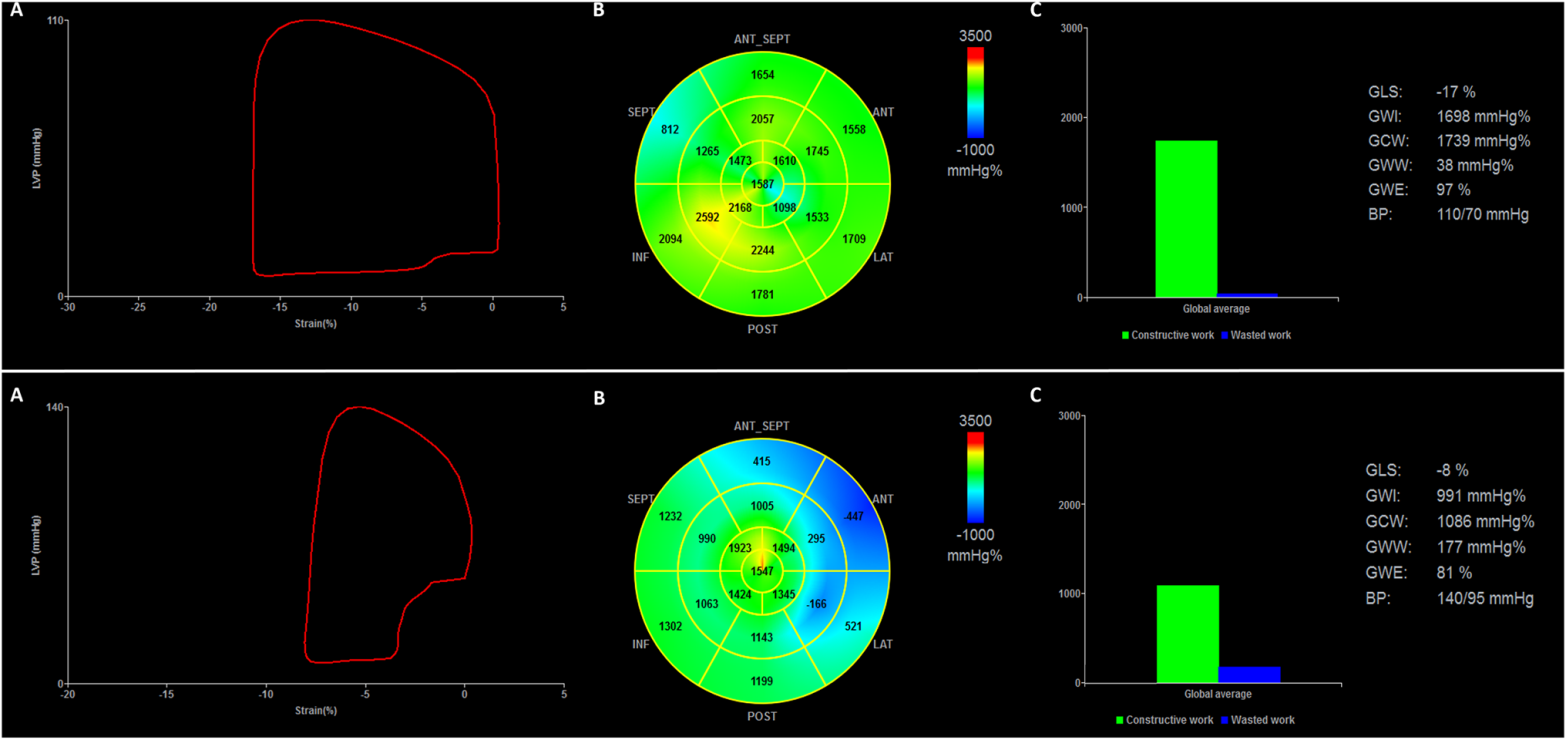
Pressure–strain loops by estimated LV pressure and LV longitudinal strain by means of echocardiography. Representative example of global myocardial work measurements from an event-free AFD patient (upper panel) and a patient with adverse event at follow-up (lower panel). a LV pressure–strain loop; b Bull’s eye plot showing segmental LV myocardial work index in a 17-segment model; c constructive work (green column) and wasted work (blue column) values. *LVP* LV pressure, *GLS* global longitudinal strain, *GWI* global work index, *GCW* global constructive work, *GWW* global wasted work, *GWE* global work efficiency, *BP* blood pressure
